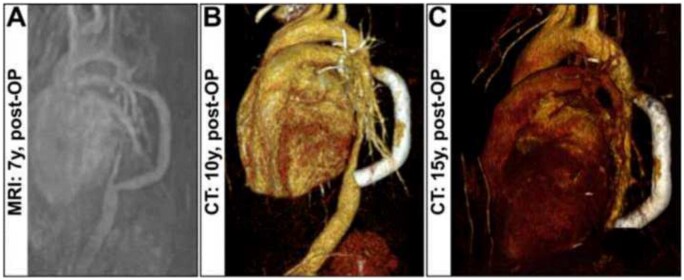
(A) Magnetic resonance image obtained at 7 years of age. (B) Computed tomography at 10 years of age. (C) Computed tomography at 15 years of age. CT: computed tomography; MRI: magnetic resonance imaging; post-OP: postoperative; y: year.
